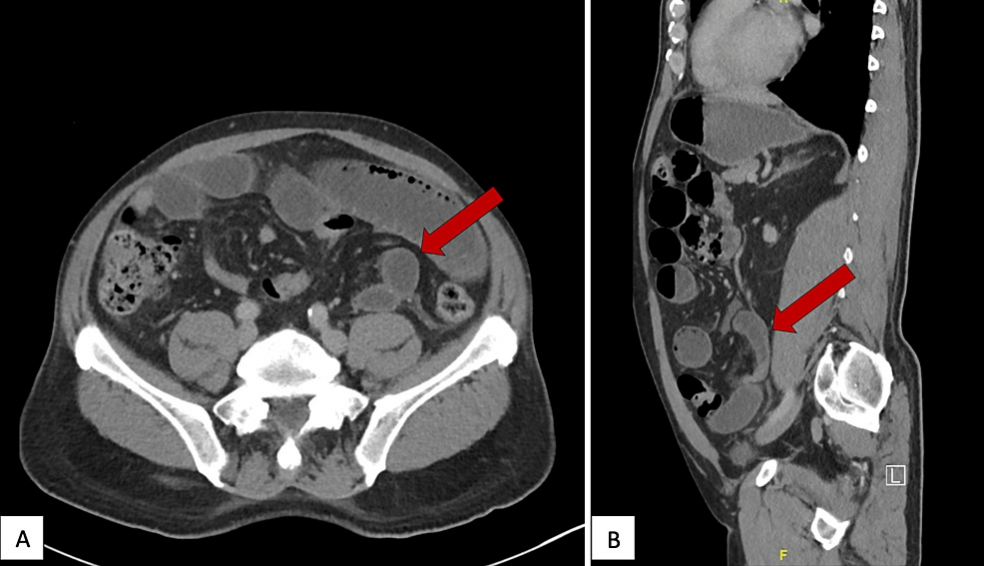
Preoperative contrast-enhanced CT taken upon admission suggested a complete or high-grade small bowel obstruction. 1A: Transverse contrast-enhanced CT with an arrow delineating encapsulated fluid-filled loops of bowel in the left lower abdomen near the sigmoid mesocolon. 1B: Sagittal contrast-enhanced CT with an arrow delineating obstruction in the left hemipelvis with proximal dilation of the small bowel.
